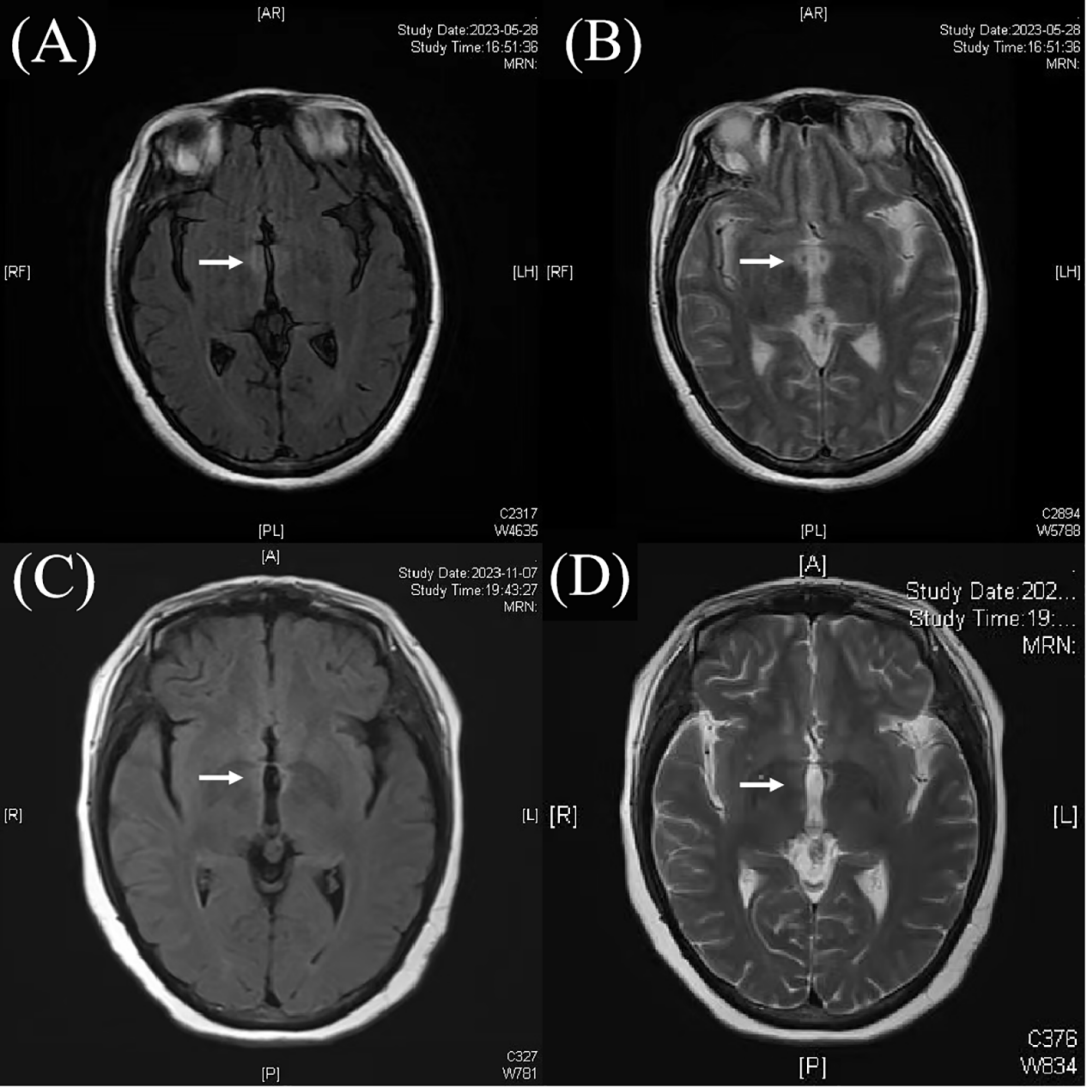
Magnetic resonance images (MRI) showed abnormal lesions around the third ventricle (A, B). After treatment, the abnormal lesions of the third ventricle was weaker than before (C, D).
